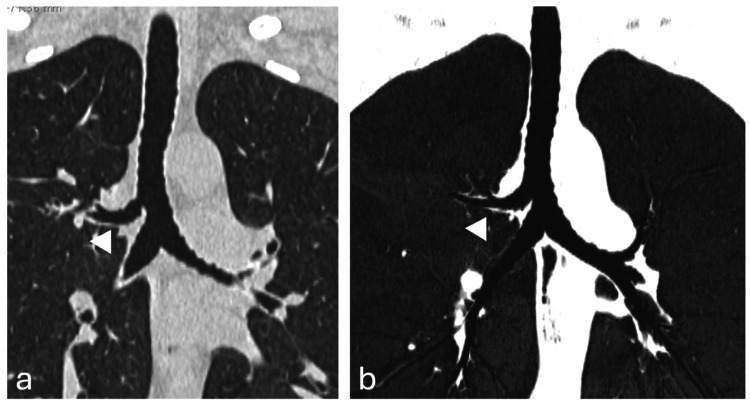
Computed tomography scan of tracheal bronchus. Coronal reformat of a chest CT scan on average (a) and minimum-intensity (b) projections depicting a tracheal bronchus (arrowheads). Image credit: Dr. Spyridon Prountzos and Prof. Efthymia Alexopoulou.
